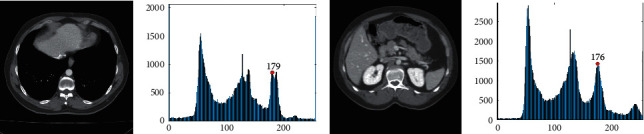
Maximum gray value of the liver (LV_M) in CT slice image. (a) The upper section. (b) LV_M of Figure 6(a), (c) The lower section. (d) LV_M of Figure 6(c).
